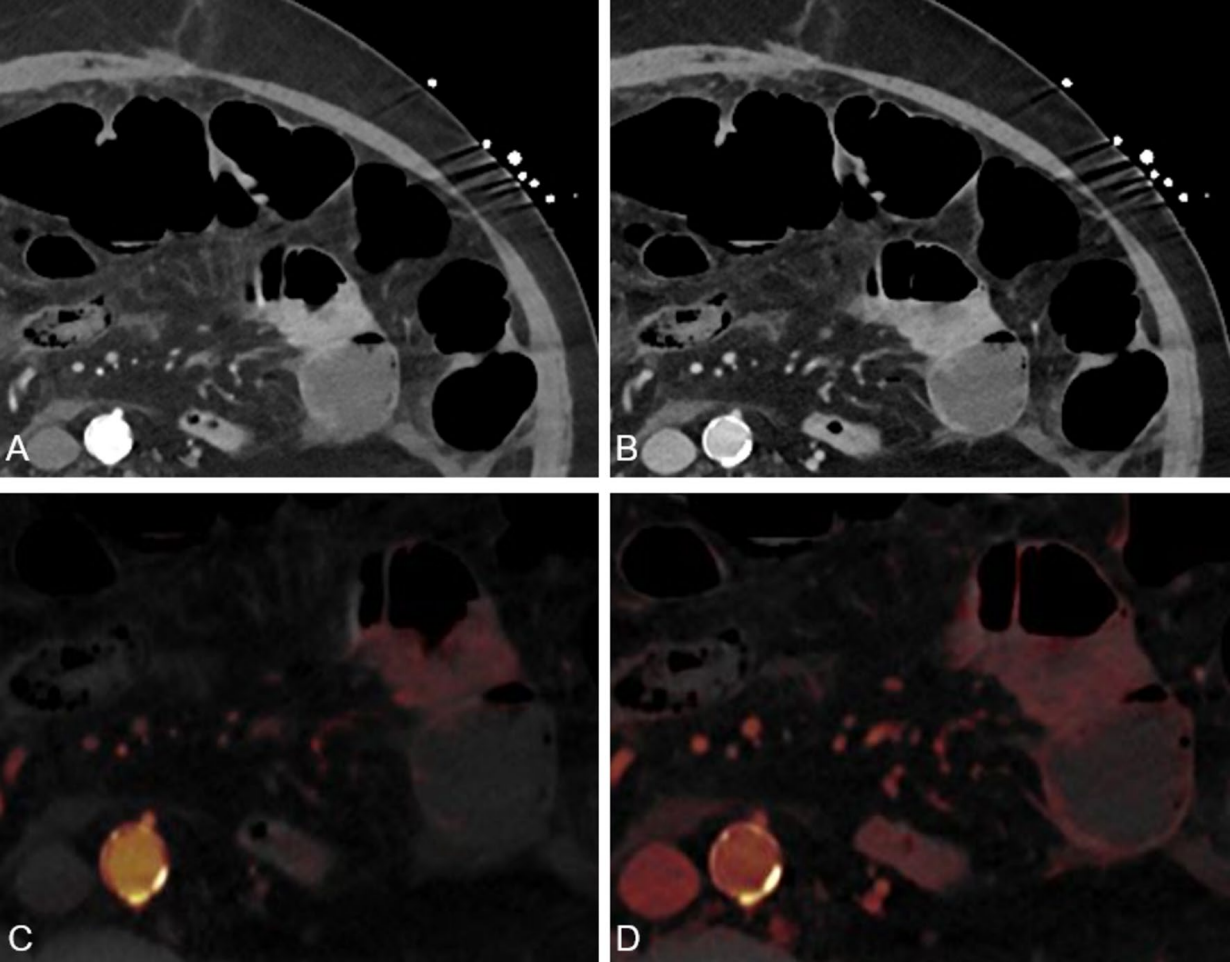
67-year-old man suffering from acute abdomen and left-sided abdominal pain. Transversal conventional late arterial (LA) phase (A) and portal venous (PV) phase. (B) CT series show slightly decreased enhancement of a small bowel loop in the left abdomen in a surgically proven case, particularly in the LA phase. At the visual analysis, LA phase iodine maps (C) were significantly better rated regarding bowel ischemia conspicuity assessment, contrast and image noise compared to PV phase iodine maps (D) (all categories, *P* < .001). While LA phase iodine maps show a distinct visual difference between ischemic and non-ischemic bowel, there is only a slight visual difference on PV phase iodine map, which makes the diagnosis challenging
